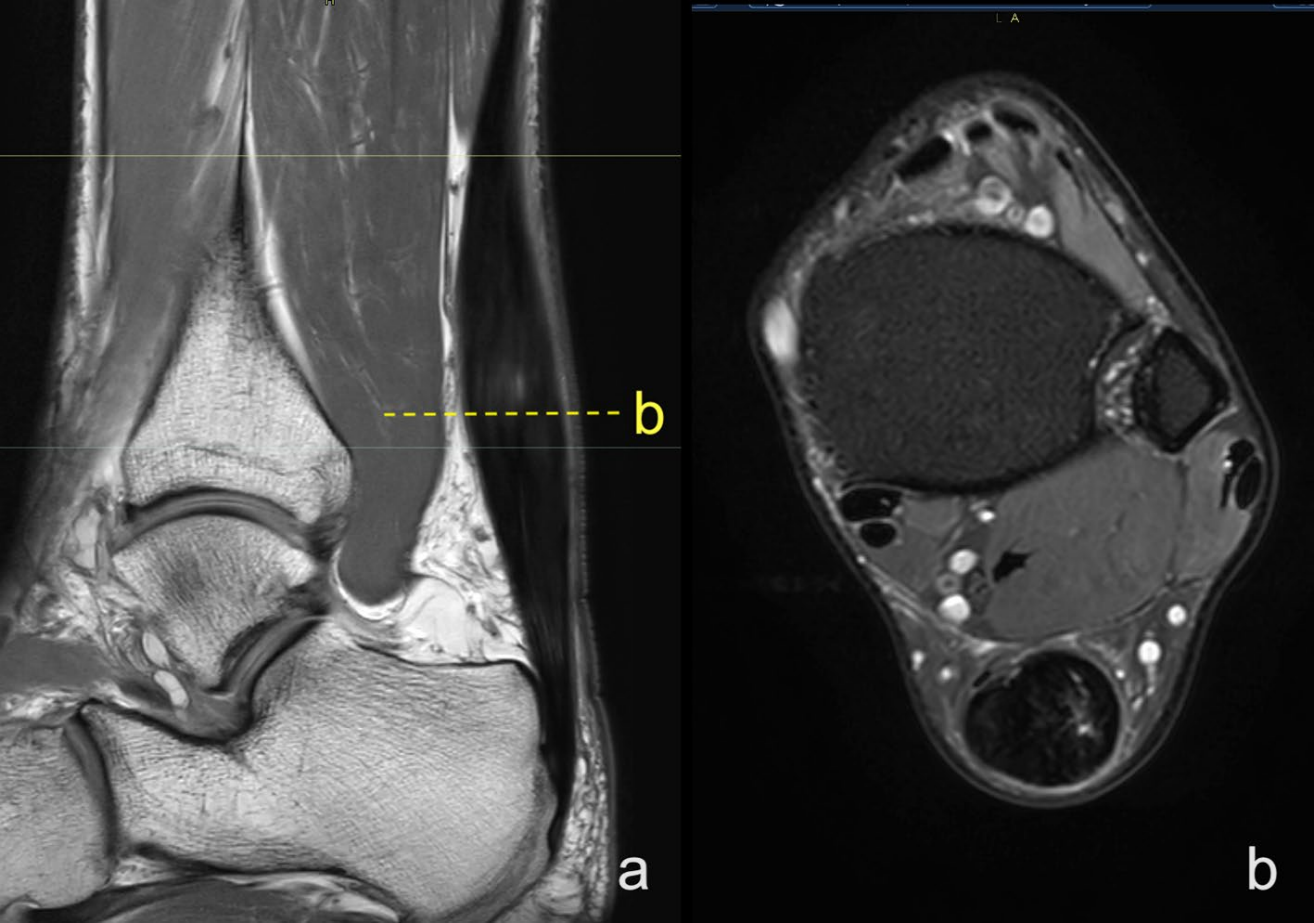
Midportion tendinopathy of the right Achilles tendon in a 45-year-old male who used to run about 3–5 km per day presenting with Achilles pain. Clinical diagnosis of tendinopathy was made with a suspicion of partial rupture. MRI showed a spindle-shaped Achilles tendon with alterations in the signal in the thickened part. (a)- Sagittal section, PD- weighted TSE, (b) axial section PD- weighted SPAIR.
